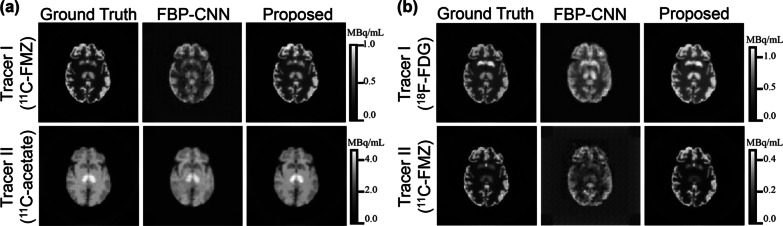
The representative reconstructed images from the tracer combination experiment. a Tracer combination I ($$^{11}$$C-FMZ/$$^{11}$$C-acetate), b Tracer combination II ($$^{18}$$F-FDG/$$^{11}$$C-FMZ). These images show the 10th frame of the dynamic images
